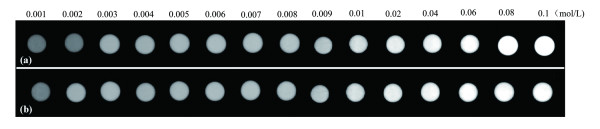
Axial CT images. {(Au^0^)_200_-G5.NH_2_} DENPs (a) and iohexol (b) at a range of concentrations in 1.5-mL microcentrifuge tubes.
